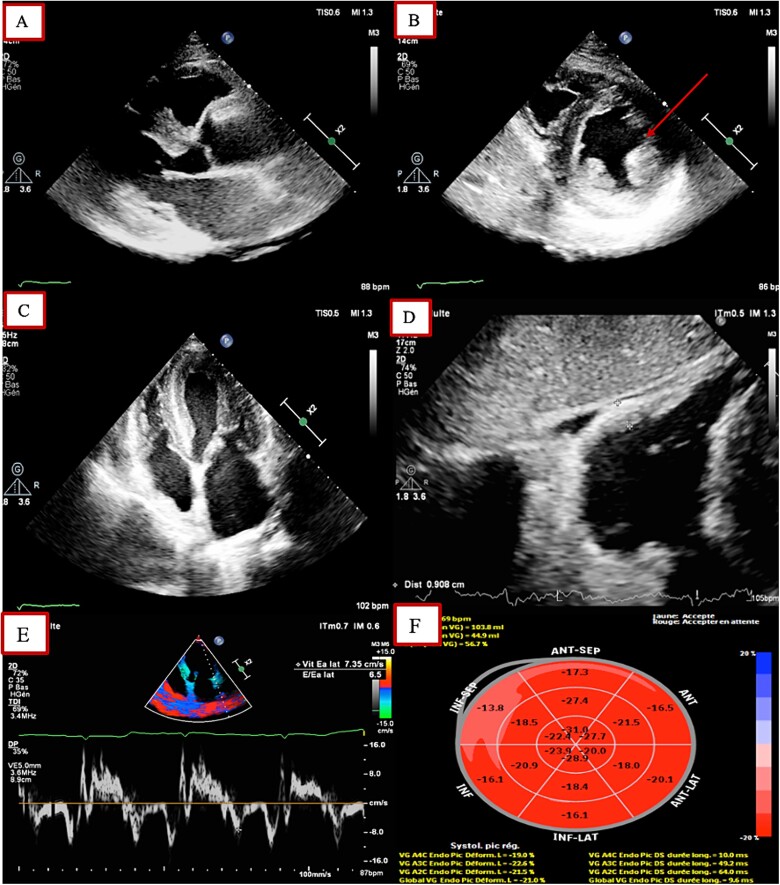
TTE findings. Parasternal long and short axis view showing concentric left ventricular hypertrophy with extension to the papillary muscles (red arrow). (C): 4 chamber view showing biventricular hypertrophy with left atrial enlargement (D): Subcostal view showing right ventricular hypertrophy. (E): Lateral E’ velocity suggesting diastolic dysfunction. (F): Normal global longitudinal strain calculated at −21%.
